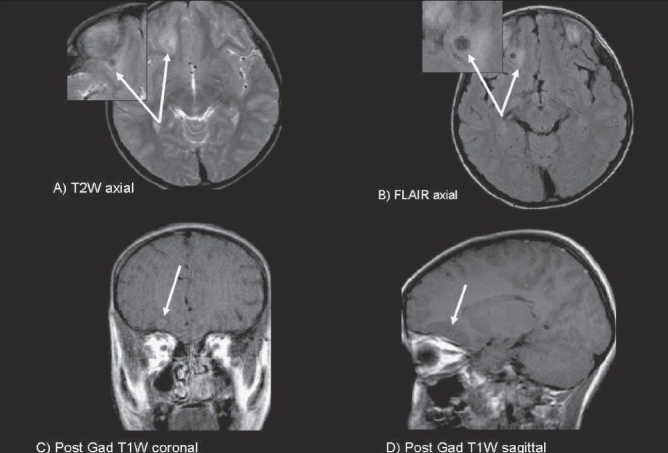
T2W [A] and FLAIR [B] images show hyperintense signal (white arrows) in the right basifrontal region with a central focal area of hypointensity. Postcontrast (gadolinium) axial (C) and sagittal (D) images reveal a ring-enhancing lesion with perilesional edema (white arrow). These findings were suggestive neurocysticercosis
